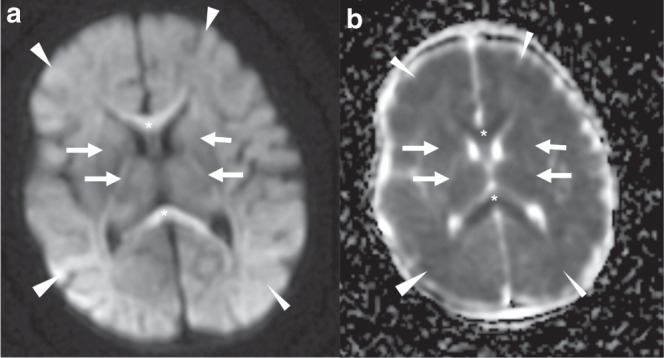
Acute, severe deep gray and watershed pattern of injury. DWI (a) and ADC map (b) demonstrate restricted diffusion involving the deep gray (white arrows denoting reduced diffusion in the putamen, globi paladi, ventrolateral thalamus, posterior limb of the internal capsule) and watershed pattern (white arrowheads denoting reduced diffusion involving the subcortical white matter), with global injury score 84. Additional reduced diffusion involving the genu and splenium of the corpus callosum (asterisks) is compatible with pre-Wallerian degeneration from intramyelinic edema.
